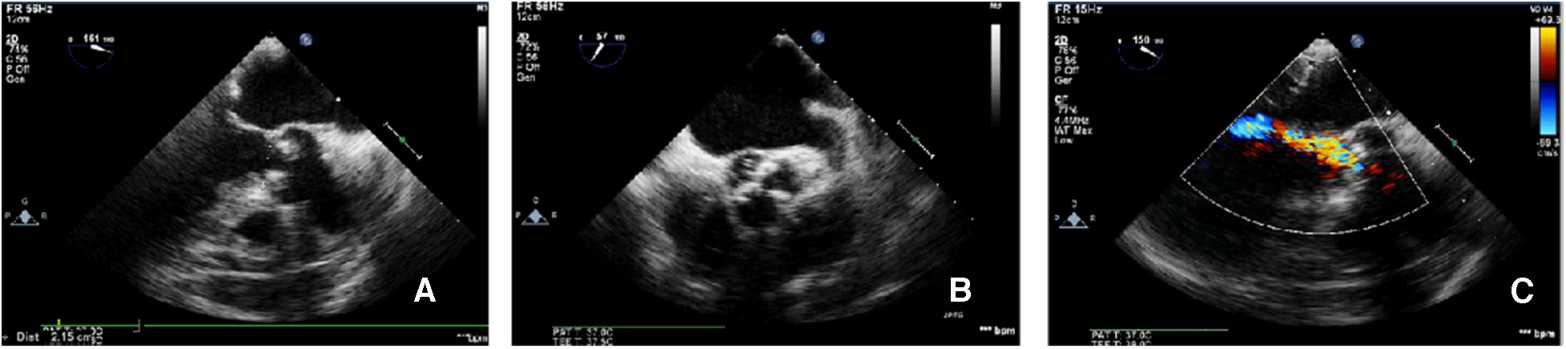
TEE-measured virtual valve annulus diameter. The TEE-measured virtual aortic valve annulus diameter was 21.5 mm (A); the tricuspid aortic valve leaflets were severely calcified (B); color Doppler ultrasound showed mild-to-moderate aortic valve regurgitation (C).
